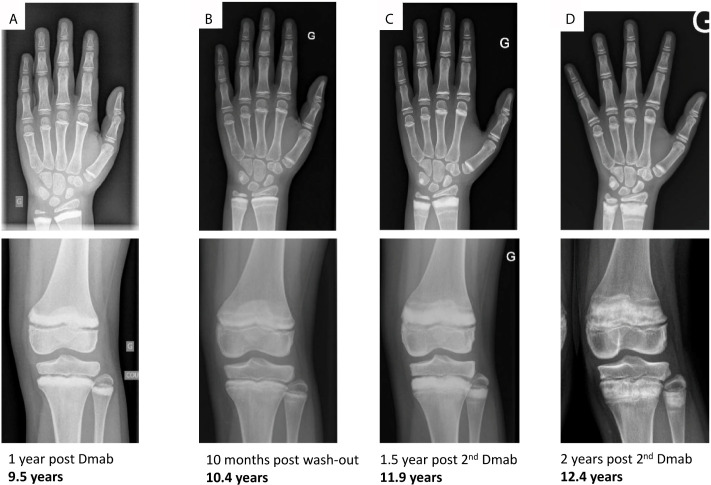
Hand and knee radiographs during follow-up. Radiographs taken (A) after 1 year of denosumab (onset of sclerotic metaphyseal bands), (B) after 10 months of denosumab wash-out (reduction of sclerotic bands), (C) after 1.5 years of the 2^nd^ course of denosumab (more pronounced sclerotic metaphyseal bands) and (D) after 2 years of the 2^nd^ course of denosumab (severe and persistent sclerotic metaphyseal bands).
